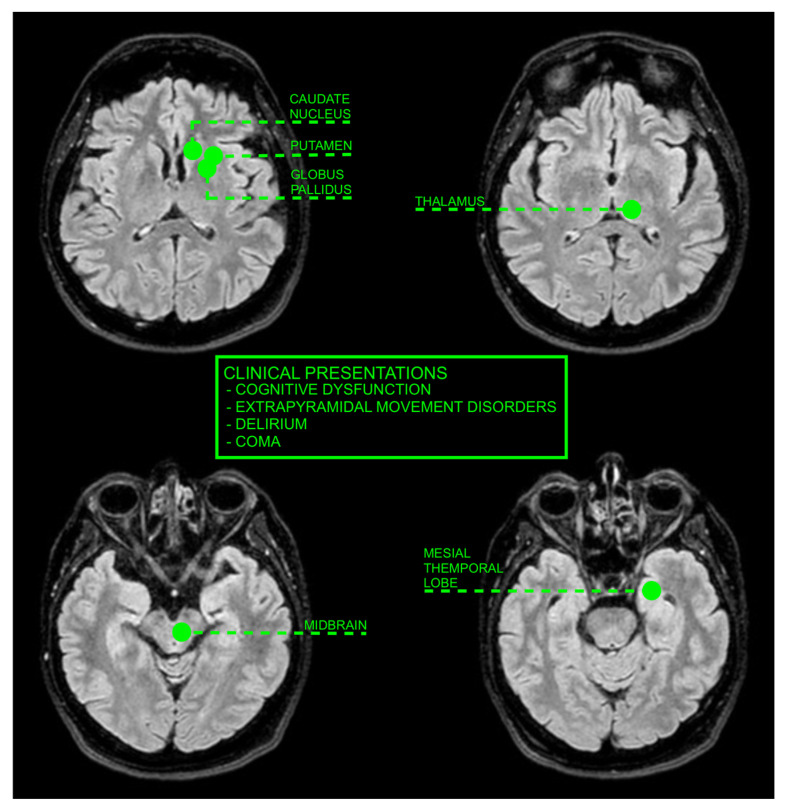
FLAIR images on the axial plane show the anatomical structures involved in UE and their main clinical presentations.
